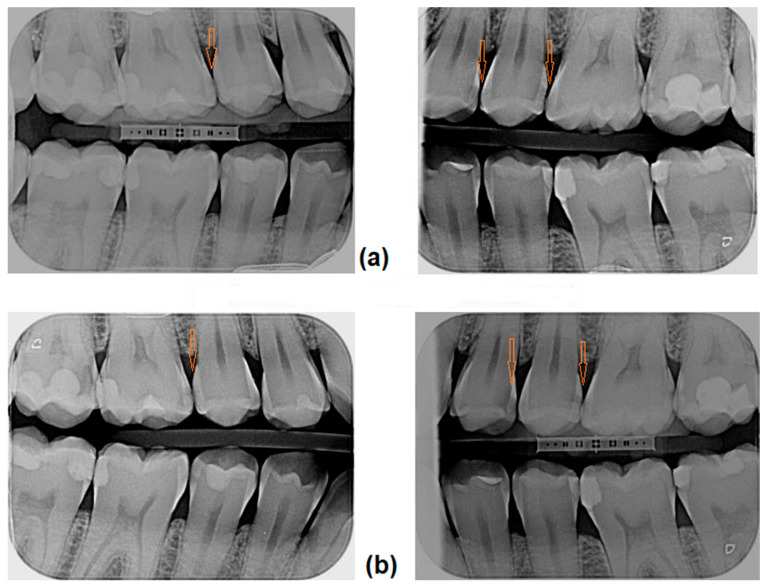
Bitewing radiographs before (a) and 1 year after treatment (b). Arrows indicate the initial proximal caries lesions and their condition at the 1-year follow-up. Tooth 16 mesially (caries D1)—infiltrated and arrested after 1 year. Tooth 25 distally (caries reaching DEJ)—remineralization, progressed after 1 year to caries D1. Tooth 24 distally (caries D1)—control (no treatment) progressed after 1 year to caries D2. E2 is caries reaching the inner half of enamel, D1 is caries reaching the outer third of dentin, and D2 is caries reaching the middle third of dentin.
